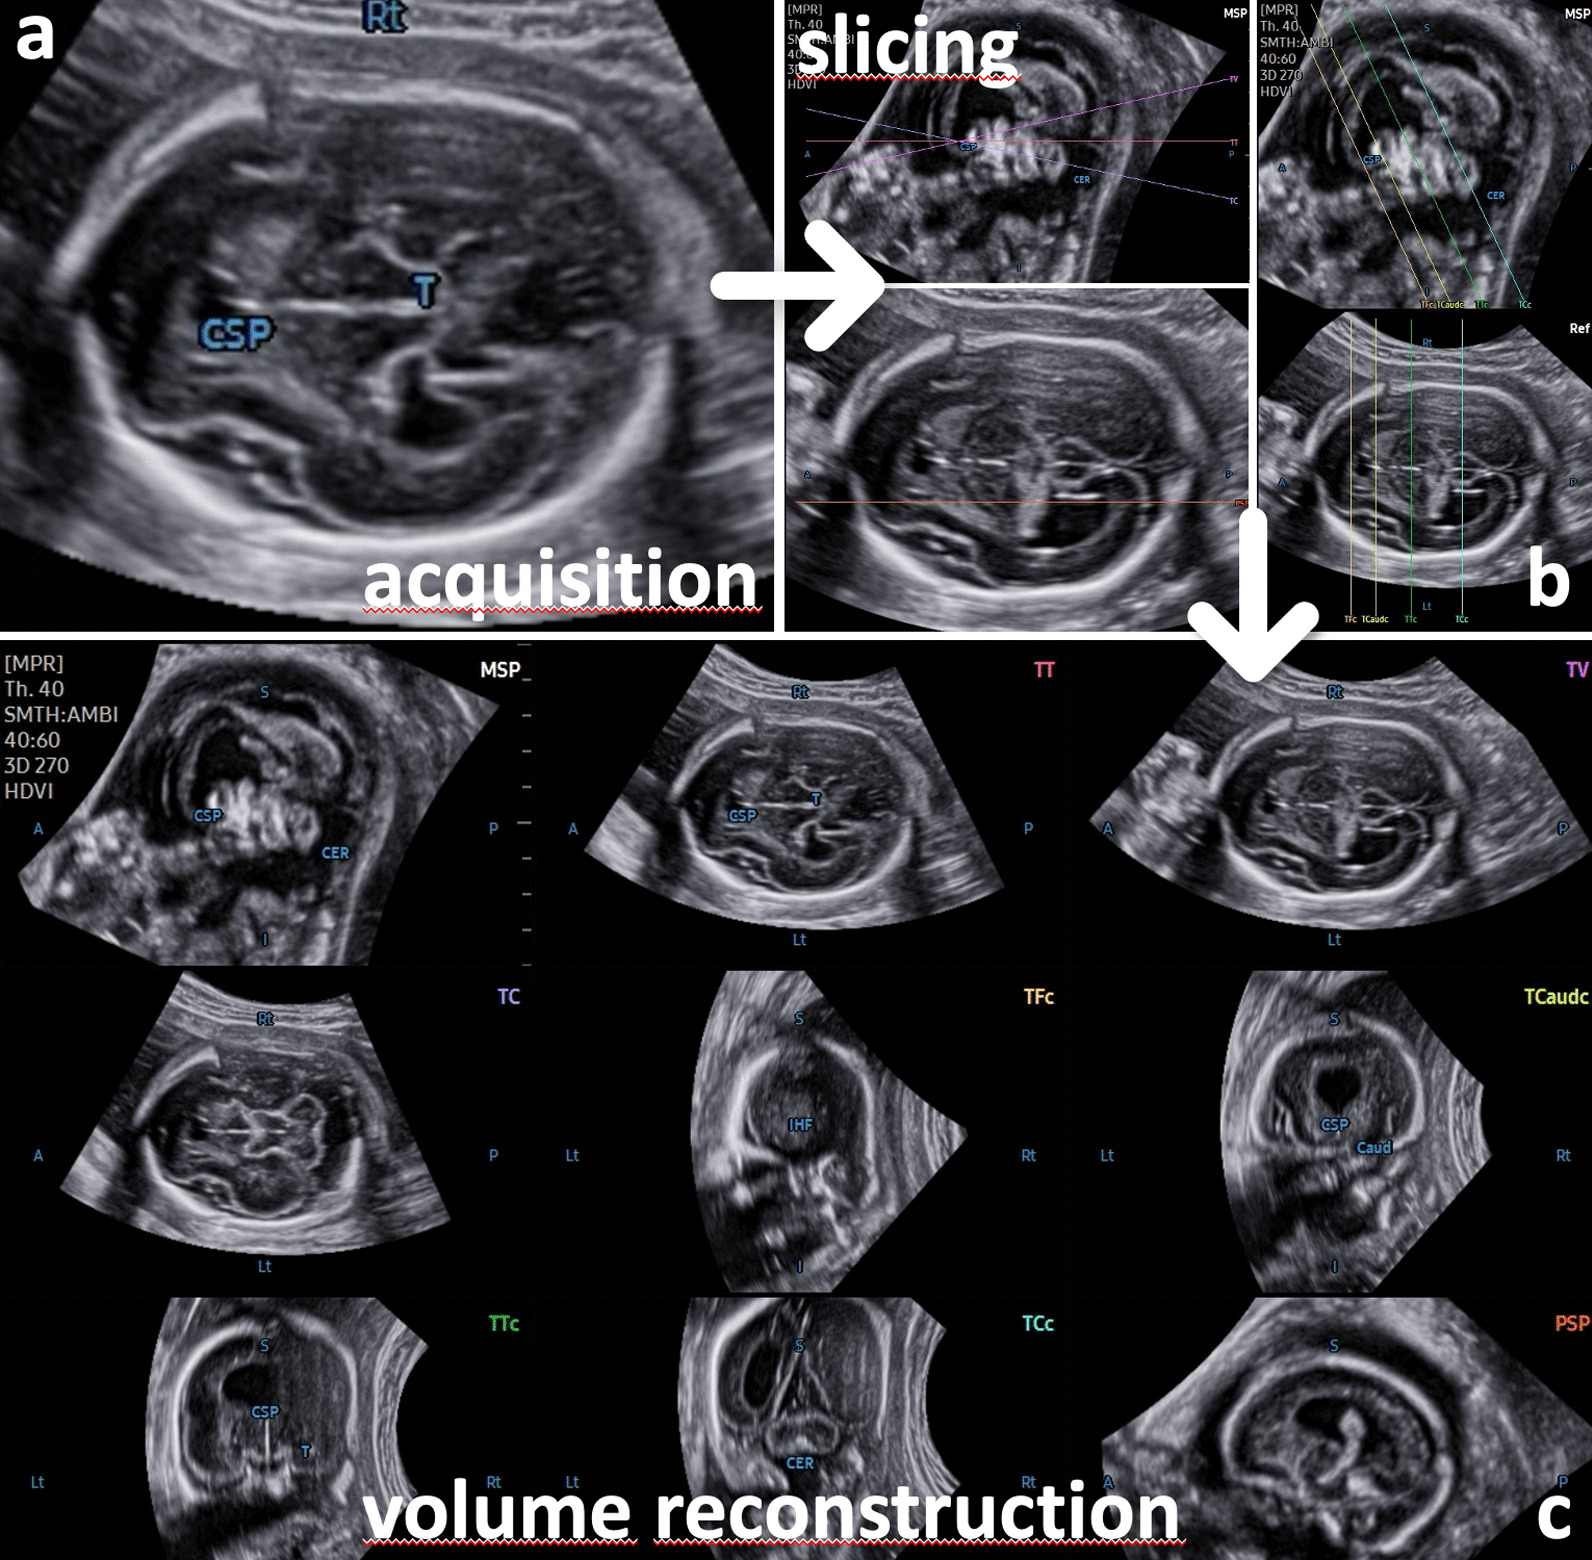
The transthalamic diagnostic plane (recommended for biparietal diameter quantification) is needed for proper volume acquisition. The part of the frontal lobe is not sufficiently delineated, and an enlargement of the lateral ventricle is suspected. For further assessment, additional views are necessary. Application of the 5D CNS+™ algorithm automatically reslices the volume data set in predefined diagnostic planes as needed for a complete neurosonogram. The reconstructed planes show abnormal CNS morphology found in errors of ventral induction (e.g., semilobar holoprosencephaly)
